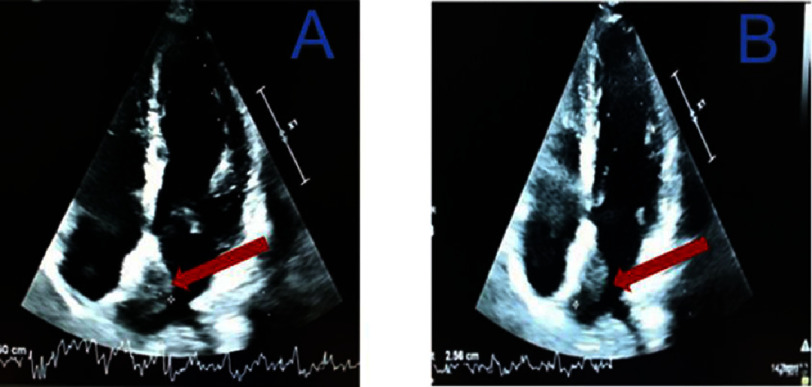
Transthoracic echocardiogram showing a left intra-atrial mass (arrow) attached to the inter-atrial septum and measuring 2.5 cm (A = horizontal diameter and B = vertical diameter).
